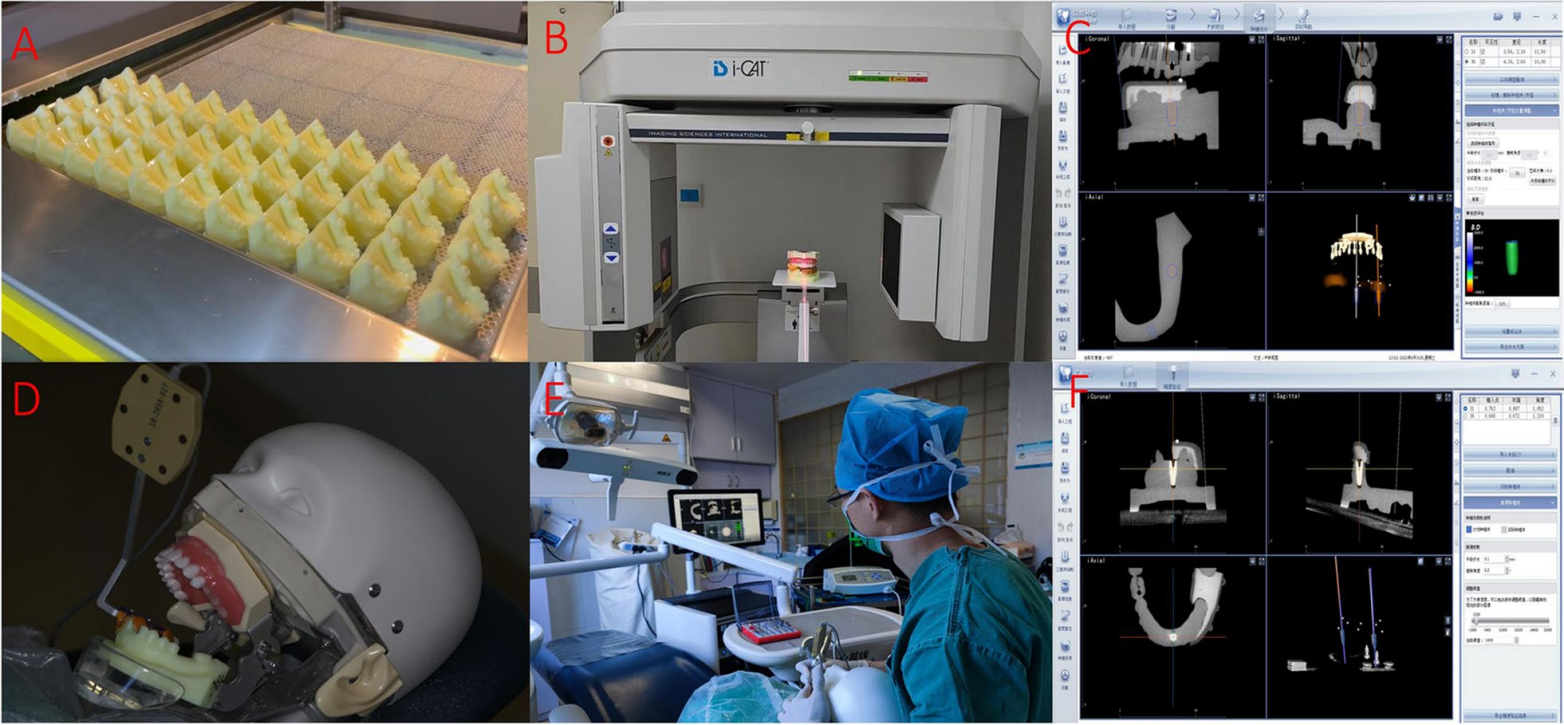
The procedure of the experiment. A Printing 3D models B Taking preoperative CT. C Designing the preoperative plan. D Fixing the model and reference plate E Implanting practice with the aid of dynamic navigation F Postoperative accuracy verification
